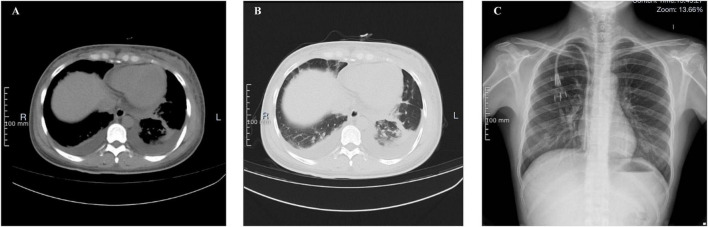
Chest imaging changes before and after treatment. (A) CT mediastinal window; (B) CT lung window; (C) chest radiograph.
